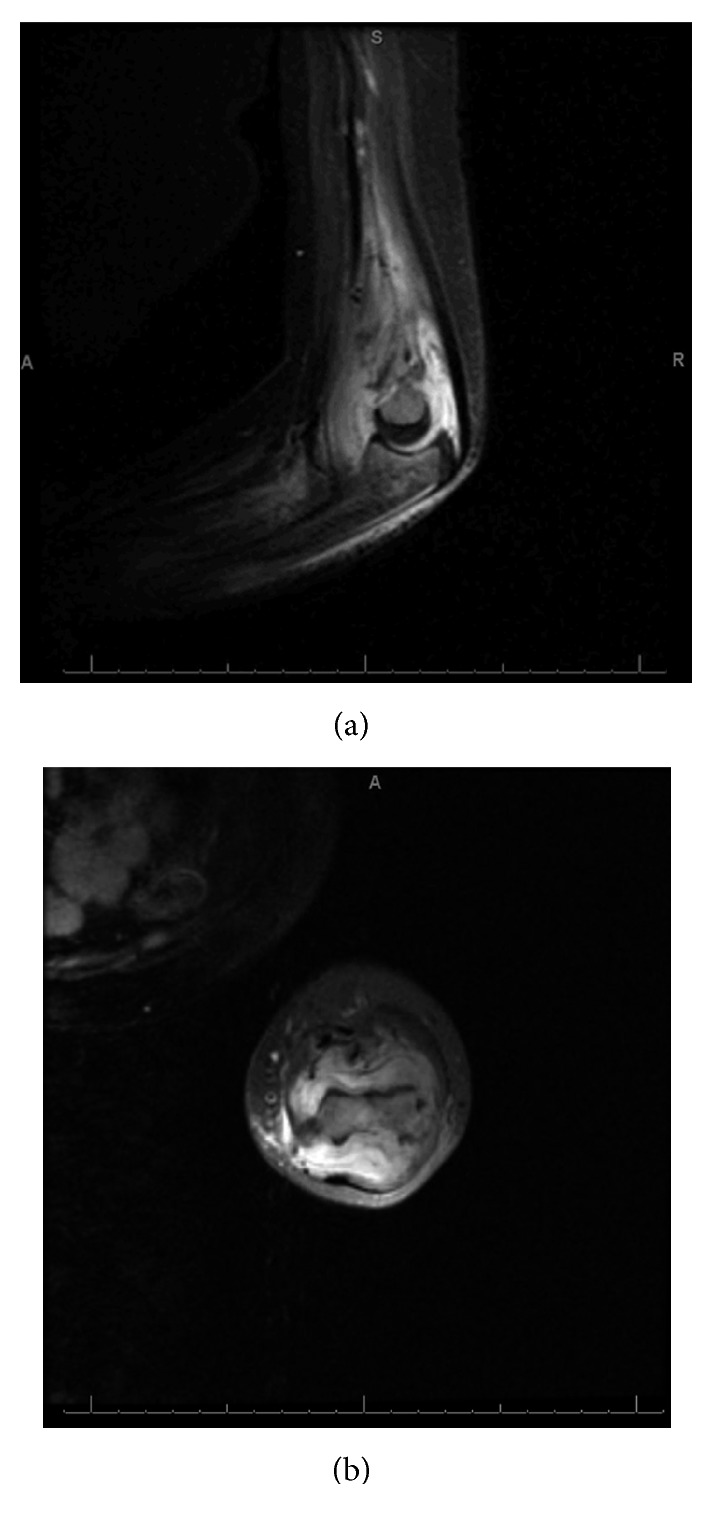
IR sagittal MRI of the left elbow showing a large loculated joint effusion with tracking up the posterior aspect of the humerus (a). T2 fat-suppressed axial MRI of the left distal humerus showing joint effusion (b).
